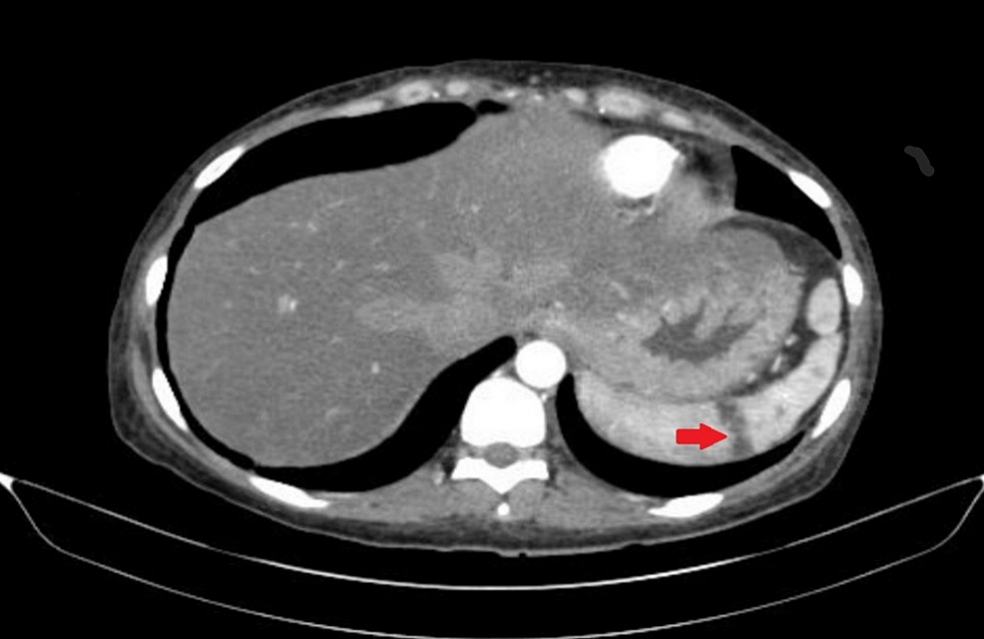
CT abdomen showing wedge-shaped splenic infarct.
